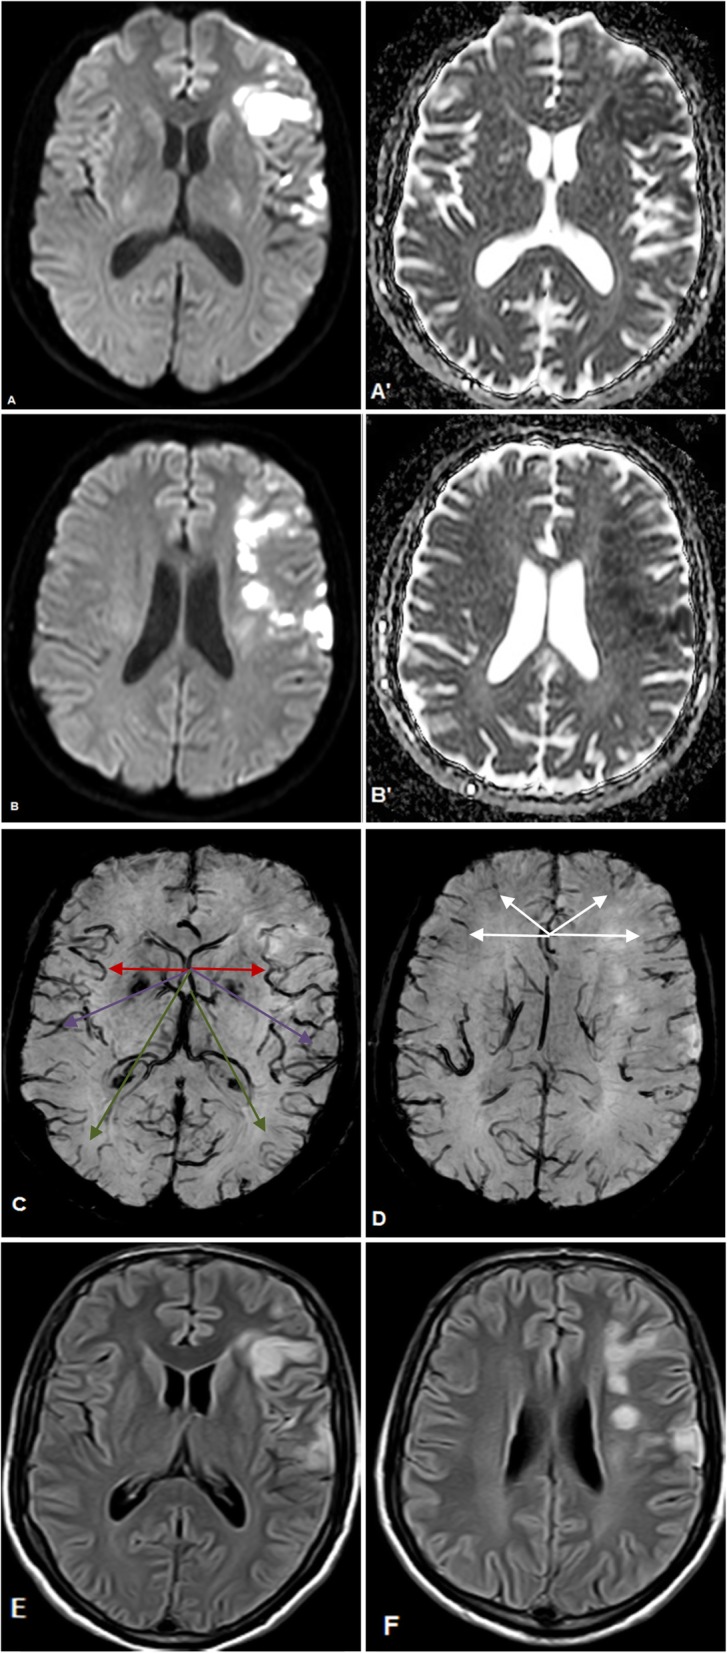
a–f 52-years-old male with left MCA territory infarct. Initial DW images and their corresponding ADC maps at the basal ganglia level (a, a') and the supraganglionic level (b, b') reveal the presence of an acute infarction in I, M1, M2, M4 and M5 regions with a resultant ASPECTS score of 5. SW images at the basal ganglia level (c) and the supraganglionic level (d) reveal the presence of APVs, in terms of either density and/or caliber and/or hypointensity, in I (red arrow) and in M2, M3 and to a milder extent in M4 regions (purple, green, and white arrows respectively) with a resultant ASPECTS score of 6 (similar colored arrows point to the corresponding areas in the contralateral normal hemisphere for comparison). DWI/SWI mismatch score is − 1. Despite the overall negative mismatch, the presence of APVs in the M3 zone, which did not show an acute infarction in the initial DW images, indicates the presence of a hidden mismatch. However, follow up FLAIR images obtained 5 days later, at the basal ganglia level (e) and the supraganglionic level (f), reveal the presence of signal alteration in precisely the same zones that showed restriction in the initial DW images with no infarct progression
